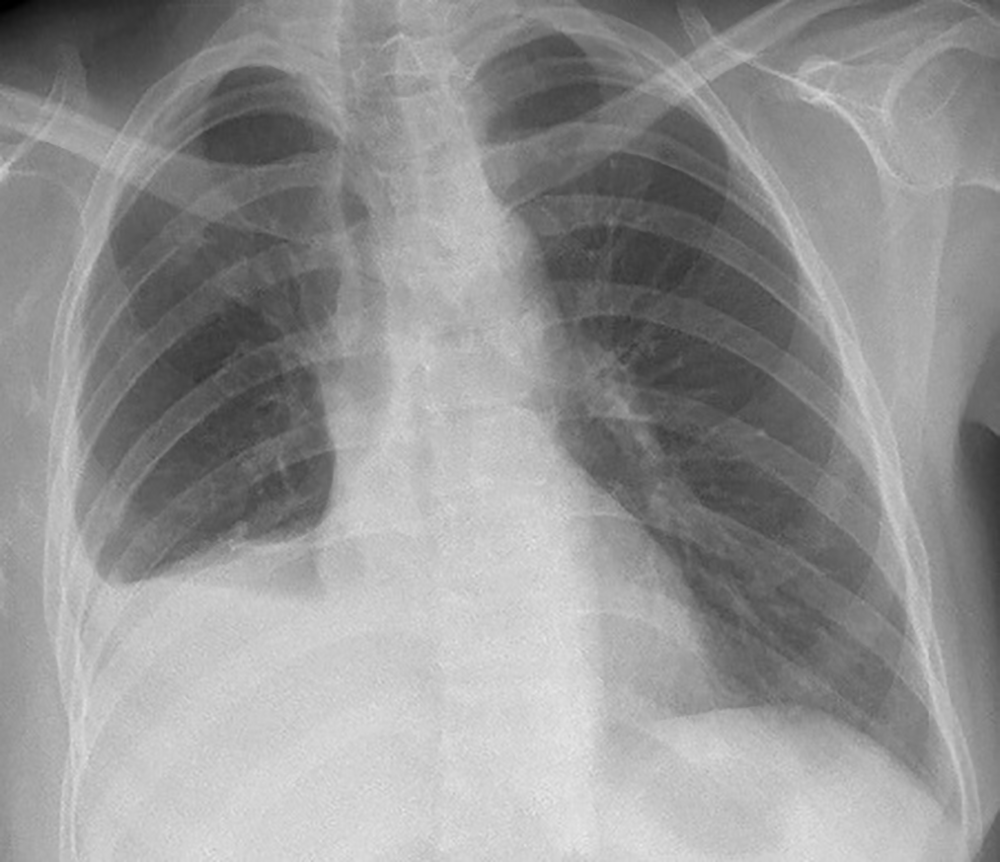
Post bilobectomy chest x-ray
